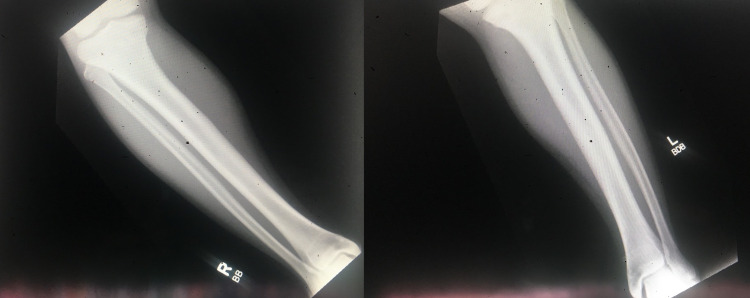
X-ray of the right and left tibia and fibula revealing tibial varus deformity.
